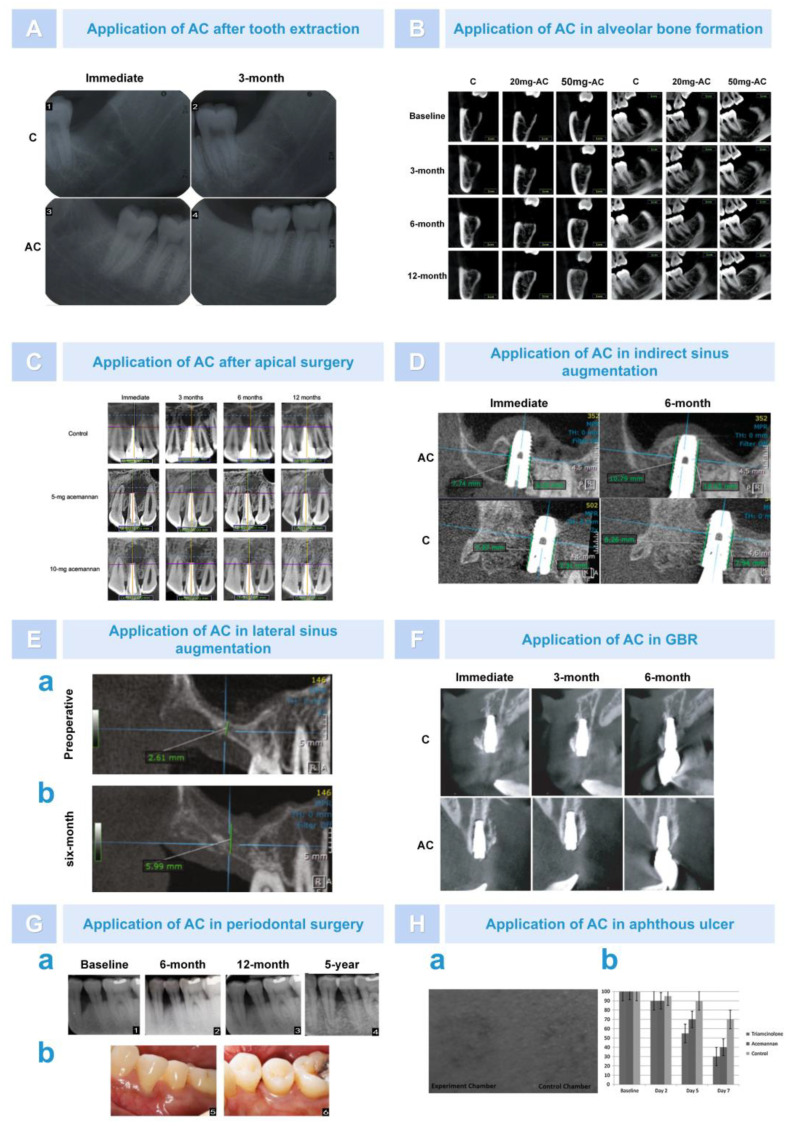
The effect of AC on bone regeneration. (A). AC reduced tooth socket volume after tooth extraction [37]. (B). CBCT showed that AC promoted alveolar bone formation after tooth extraction [101]. (C). AC induced rapid early osseous defect healing after apical surgery [102]. (D). AC enhanced endo-sinus bone formation greatly in indirect sinus augmentation [34]. (E). AC significantly increased bone height in lateral sinus augmentation surgery. The CBCT images of sagittal view at preoperation (a) and six months postoperation (b) [105]. (F). AC enhanced the dimensional stability of the regenerated tissue [107]. (G). AC induced periodontal tissue regeneration during periodontal surgery. AC increased radiographic bone fill (a) and improved the clinical appearance of the treated tooth (b) [110]. (H). AC was effective in the treatment of an aphthous ulcer. AC reduced ulcer size (a,b) [111].
